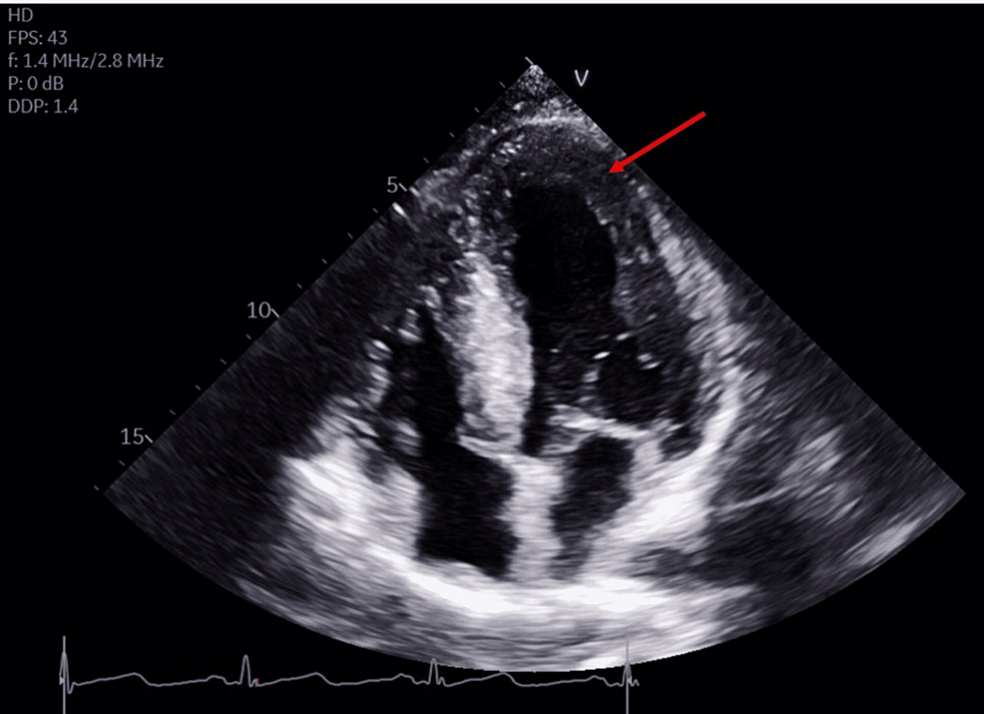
Transthoracic echocardiogram Transthoracic echocardiogram (TTE) in apical four-chamber view shows severe concentric left ventricular hypertrophy (LVH) (arrow). The ejection fraction was 60-65%. All segments contract normally. The diastolic filling pattern indicates impaired relaxation and elevated left ventricular end-diastolic pressure.
